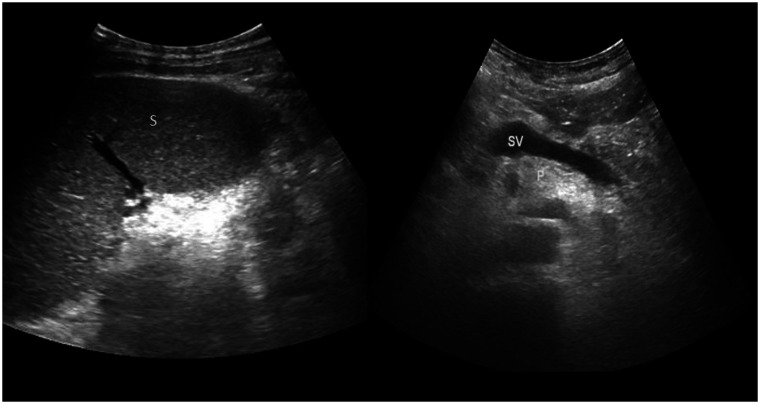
60 years old male who underwent LDLT due to fulminant liver failure and was performed ERCP due to biliary stenosis. Ultrasound image shows increased echogenicity of pancreas parenchyma compared with spleen. P, pancreas; S, spleen; SV, splenic vein.
